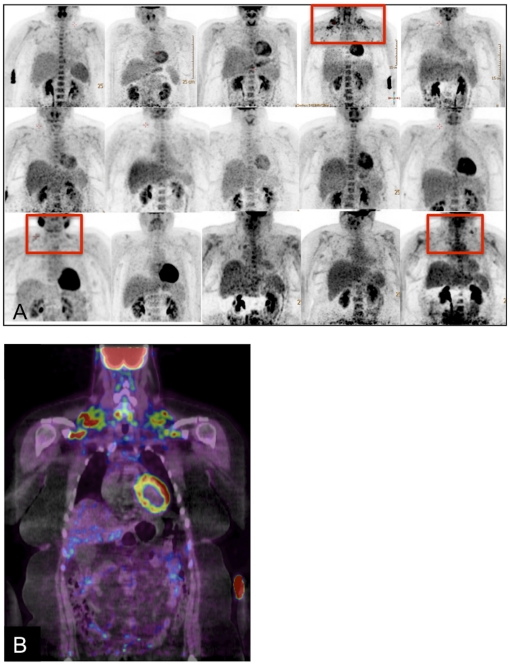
PET-images of fifteen morbidly obese subjects. Three female subjects showed BAT activity in the supraclavicular area, highlighted by a red rectangle (A). Combined PET- and CT-imaging shows FDG-uptake in supraclavicular adipose tissue (this was the morbidly obese subject that showed the most BAT activity) (B).
